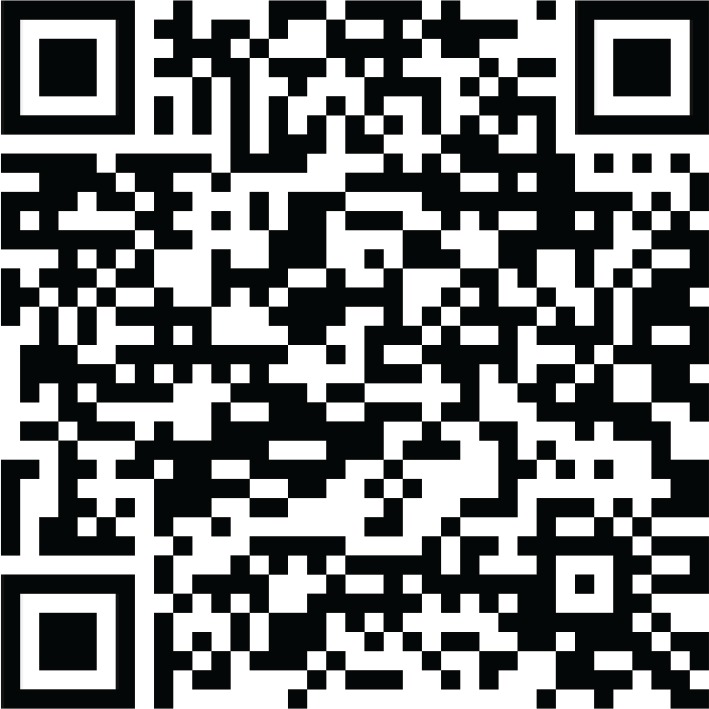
Magnetic resonance imaging, left ventricular shortaxis cine imaging: left ventricular anterior wall disruption and left ventricular pseudoaneurysm.
